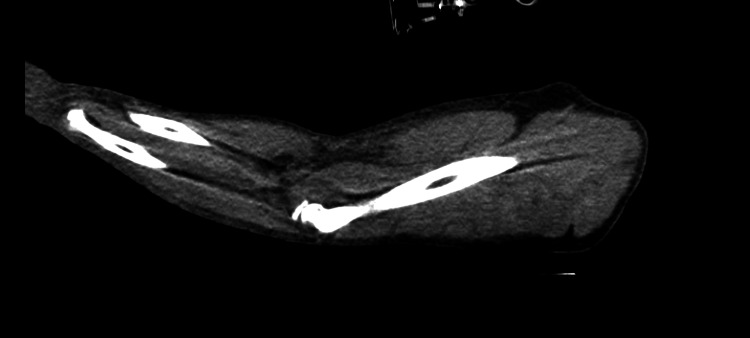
CT image of the left upper limb showing increased density of the subcutaneous tissues consistent with circumferential edema of the arm and forearm CT: computed tomography
